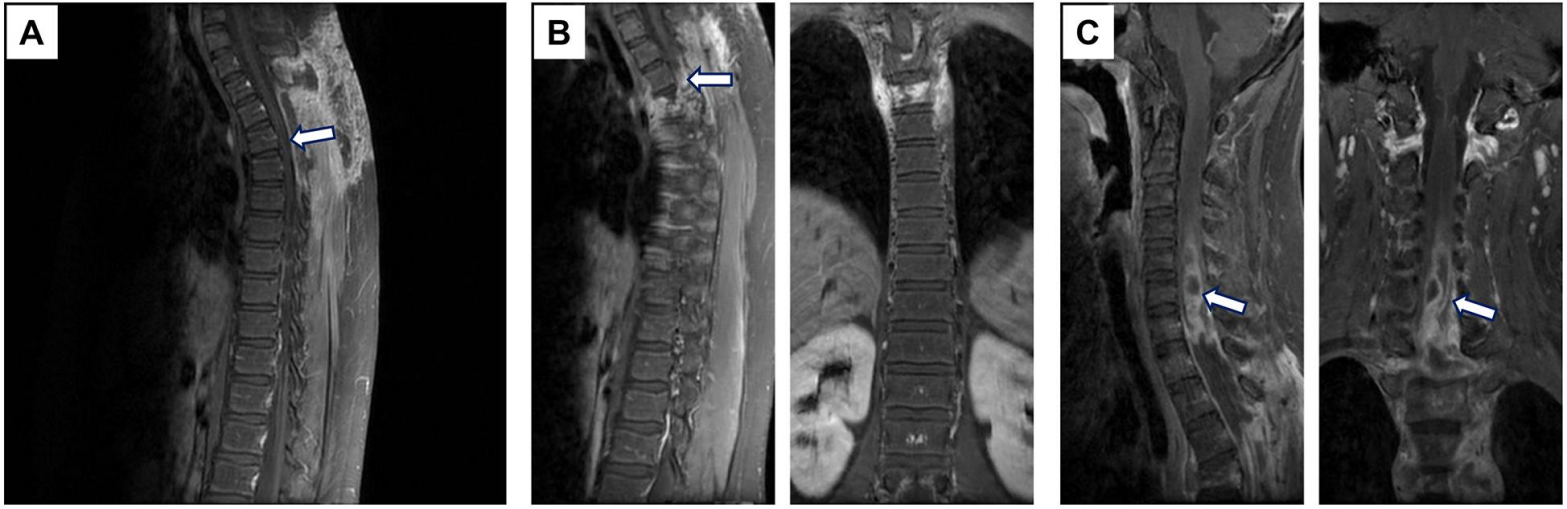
MRI imaging after treatment. (A) After the second cycle of chemotherapy, MRI showed no detectable signs of recurrence. (B) Due to poor response to chemotherapy, radiotherapy was initiated. However, tumor recurrence was observed, along with vertebral body fracture and spinal cord compression. (C) Evidence of intramedullary metastasis was noted on subsequent imaging. Arrows indicate the locations of the lesions.
